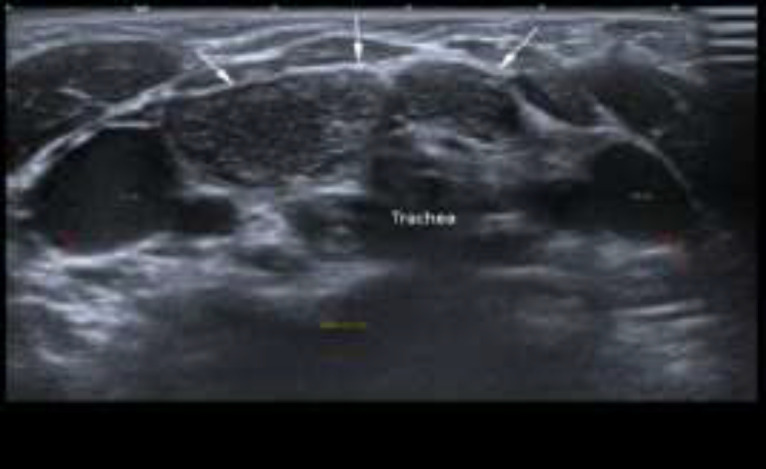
Ultrasound scan of the neck taken exactly at the same level as Figure 2a when patient was crying shows typical “starry sky appearance” suggestive of ectopic thymus (as shown by white arrows) at suprasternal region with patent bilateral internal jugular veins (as shown by red arrows).
